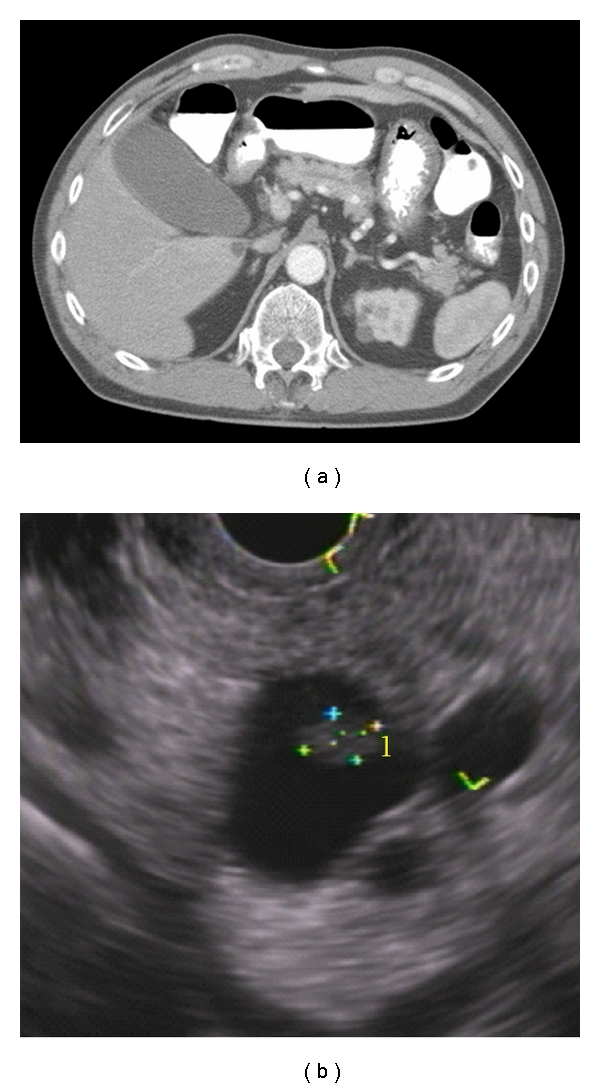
Branch-duct intraductal papillary mucinous neoplasm. (a) CT and (b) EUS showing associated mass (cross marks).
